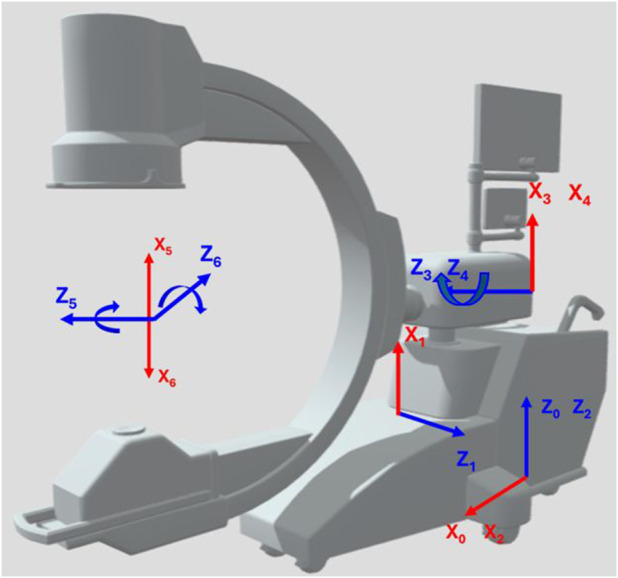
Joints of a C-arm fluoroscope. We modeled the C-arm using the manufacturing specifications of the GE Healthcare OEC 3D C-arm. Adapted from C Arm Xray Device by laurentiubodisteanu, licensed under CC BY 4.0, source: https://sketchfab.com/3d-models/c-arm-xray-device-08e65d9a5caa4dcf9338e1b980a9ec00
